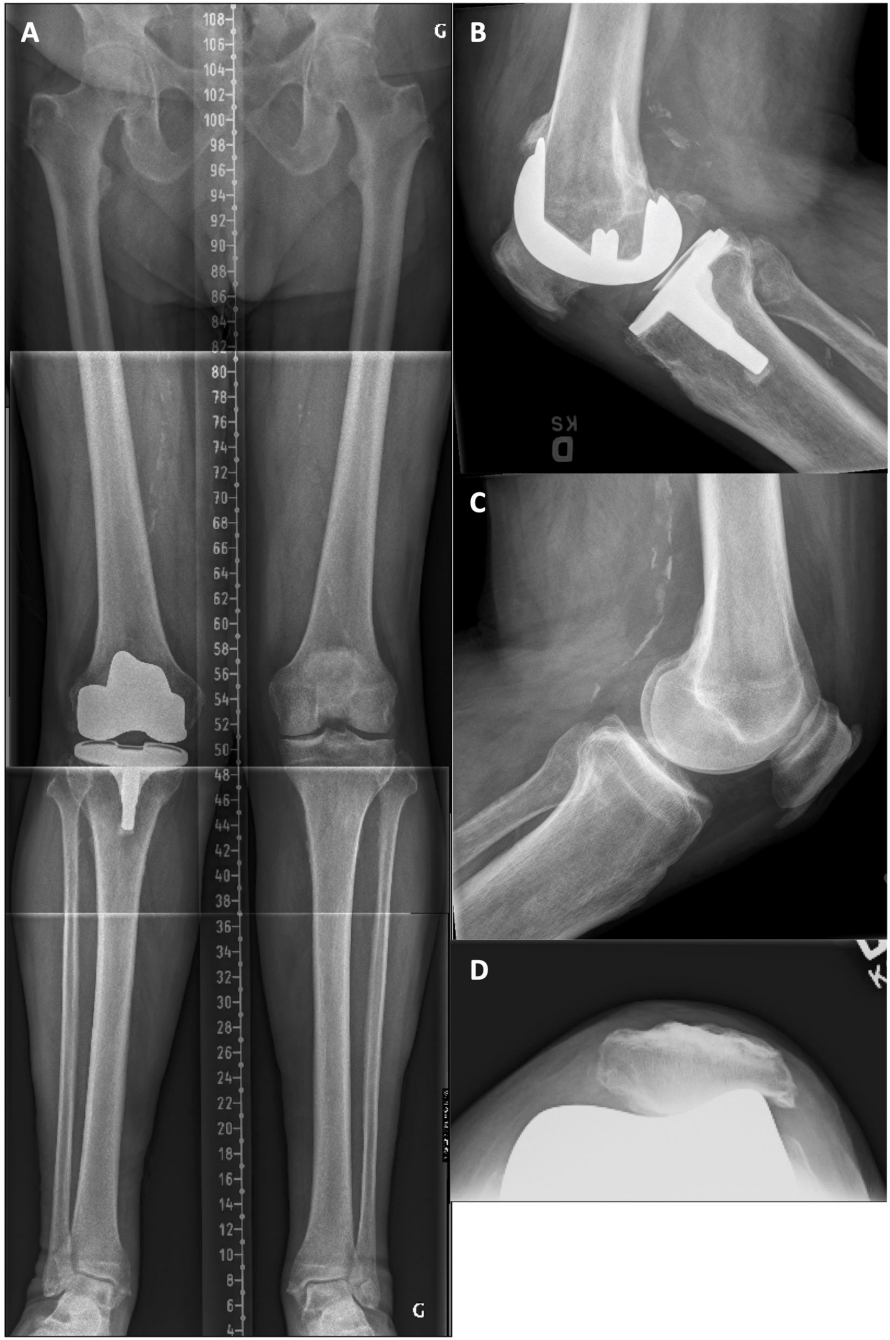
(A) Pre-revision long leg standing X-Ray reveals a right implant mDFA of 4° valgus and an mPTA of 1.5° valgus (5.5° valgus aHKA). On the intact left side, the mDFA is 2.0° valgus and mPTA 0.5° varus (aHKA of 1.5° valgus). (B) Right knee lateral view where the implant tibial slope is 1.5° anterior. (C) Left knee lateral view where the native tibial slope is 8.0° posterior. (D) Right knee skyline view showing a subluxed and worn patella.
